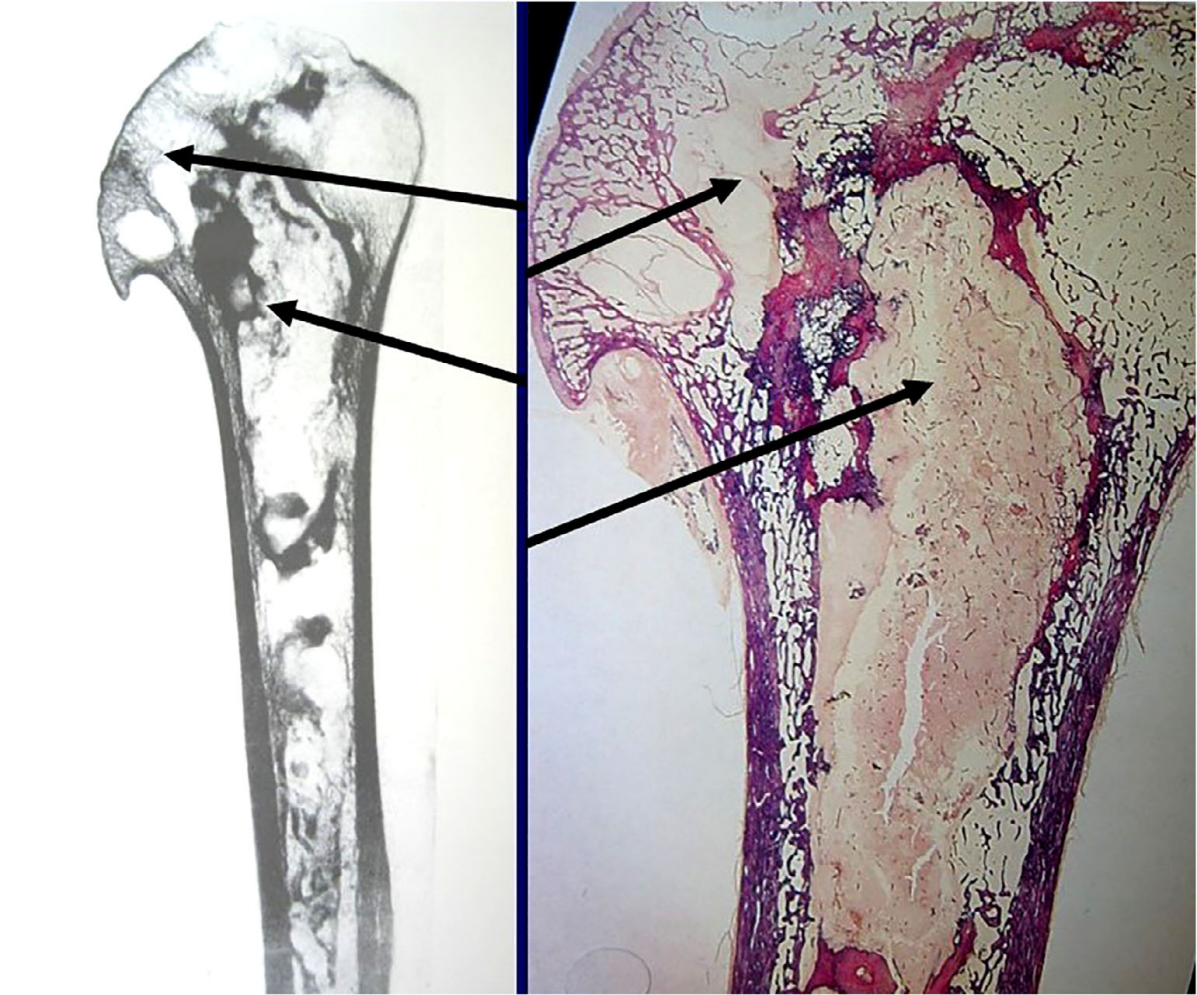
Shoulder osteonecrosis in a diver with a fatal decompression accident of: post mortem images (radiograph and anatomy) of osteonecrosis (arrows) in the proximal humeral head.
